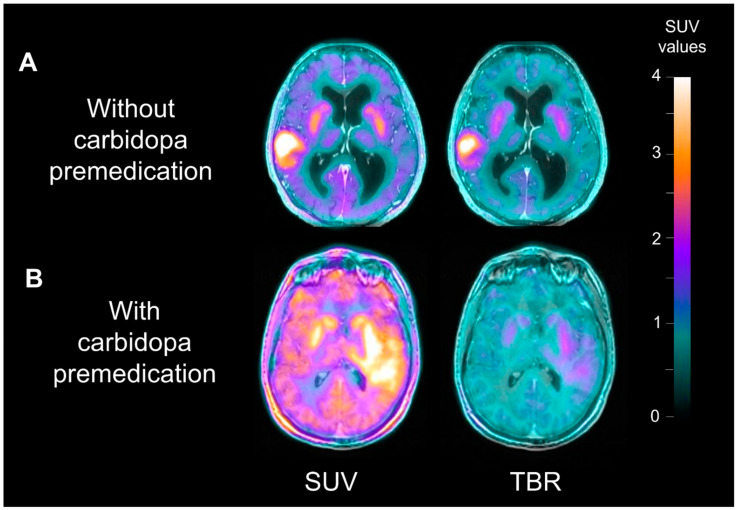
Representative examples of two patients with or without carbidopa premedication, before and after using tumor-to-healthy-brain ratio parametric images. Patient (A) is a 66-year-old woman with a newly diagnosed IDH wildtype glioblastoma (SUV_mean_ 3.2). Patient (B) is a 32-year-old man with a newly diagnosed IDH wildtype glioblastoma (SUV_mean_ 4.1). The two tumors are clearly different on TBR-parametric images, even though they have essentially the same SUV values on SUV-parametric images. Note the higher SUV uptake of striatal and healthy brain areas on B due to the carbidopa premedication.
